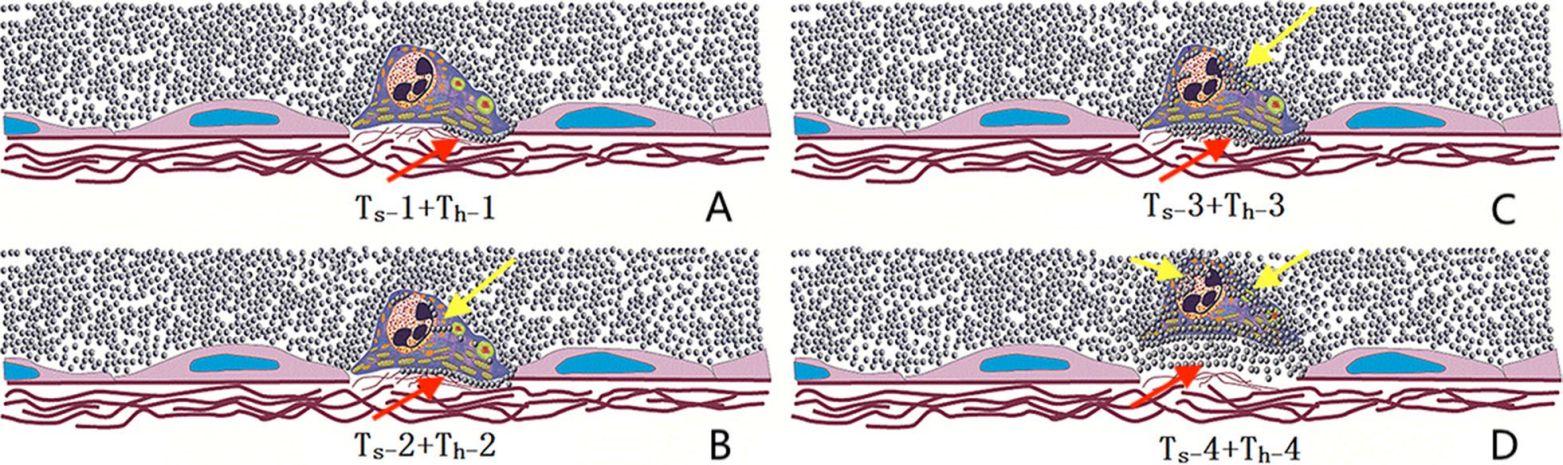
Standard application of contrast-enhanced ultrasound scoring system. a T_s_ 1: the contrast medium enters less than half of the thrombus base, and less than 3 mm in contrast medium width (red arrow); T_h_ 1: no contrast agent was found on the surface of the thrombus. b T_s_ 2: the contrast medium enters more than half of the thrombus base, but less than 3 mm in contrast medium width (red arrow); T_h_ 2: a small amount of dot-like contrast agent was seen on the surface of the thrombus (yellow arrow). c T_s_ 3: a wide gap (> 3 mm) of ultrasound contrast agent could be seen in most of the substrate (red arrow); T_h_ 3: strip-like contrast agent was seen on the surface of the thrombus (yellow arrow). d T_s_ 4: no or narrow/thin substrate (red arrow); T_h_ 4: a large amount of the contrast agent entered the surface of the thrombus (yellow arrow)
